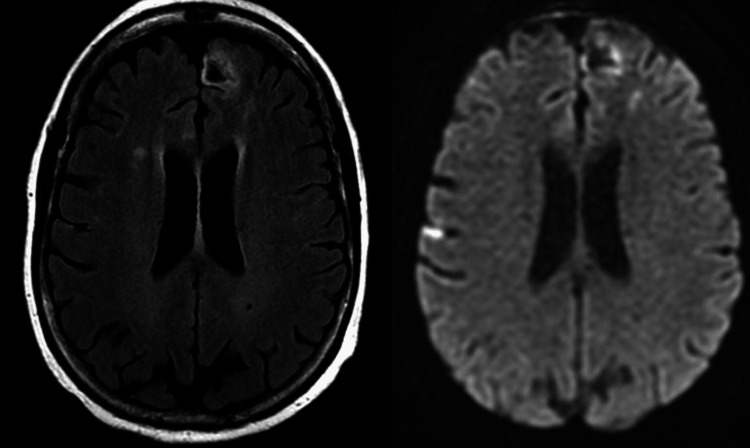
Magnetic resonance imaging (MRI) without contrast 10/28/2022 showing acute ischemic stroke Left: fluid-attenuated inversion recovery (FLAIR). The MRI shows that the largest diffusion restriction focus is 3.5 cm in the left frontal lobe. Right: diffusion-weighted image (DWI) MRI showing corresponding 3.5 cm diffusion restriction in the left frontal lobe.
